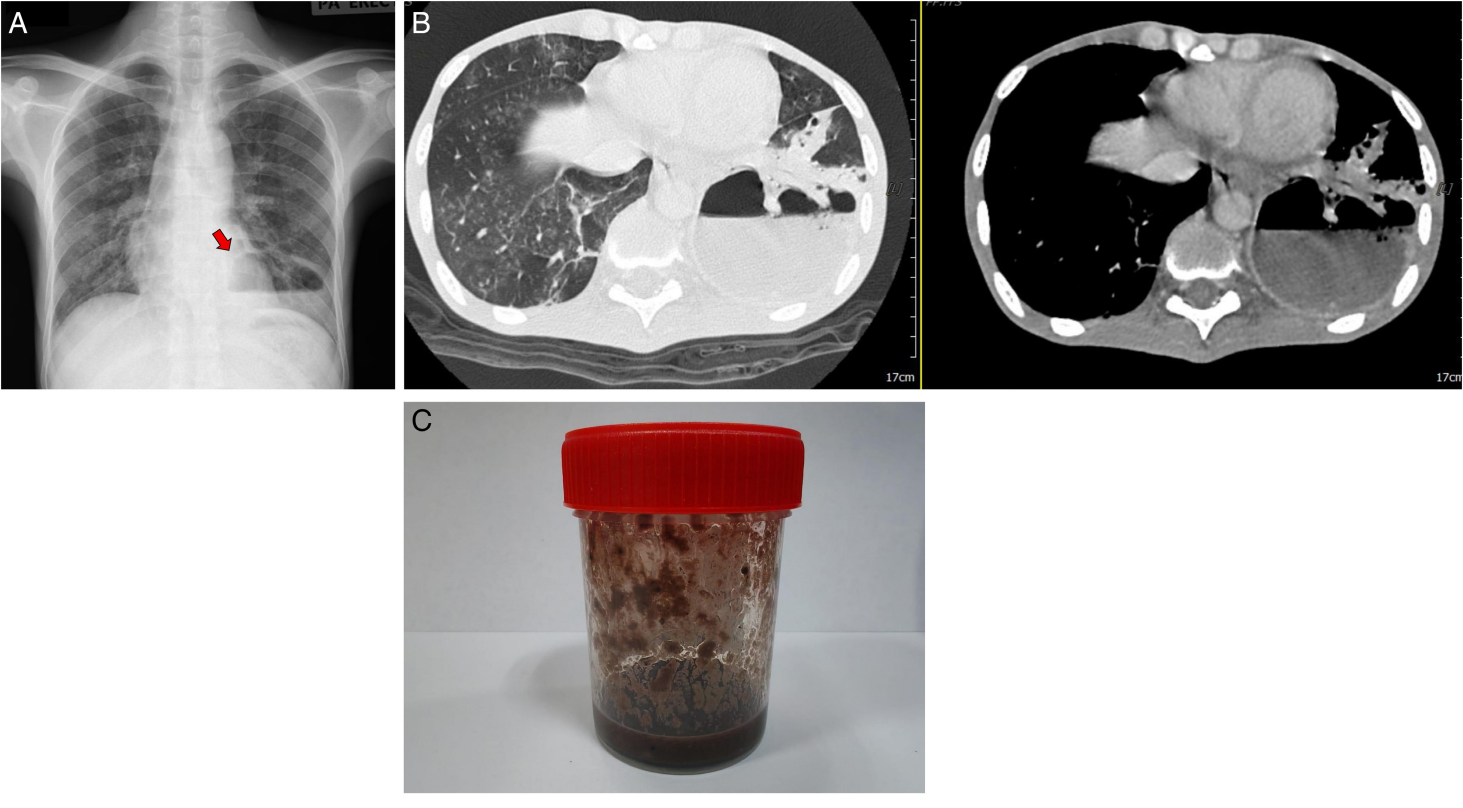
(A) Chest radiograph demonstrating a large cavity with an air–fluid level in the left lower lobe. (B) Contrast‐enhanced CT showing a thick‐walled 6 × 8 × 13 cm abscess cavity with surrounding consolidation and bilateral ground‐glass opacities. (C) Black, viscous necrotic aspirate obtained from the cavitary lesion; fungal culture yielded
